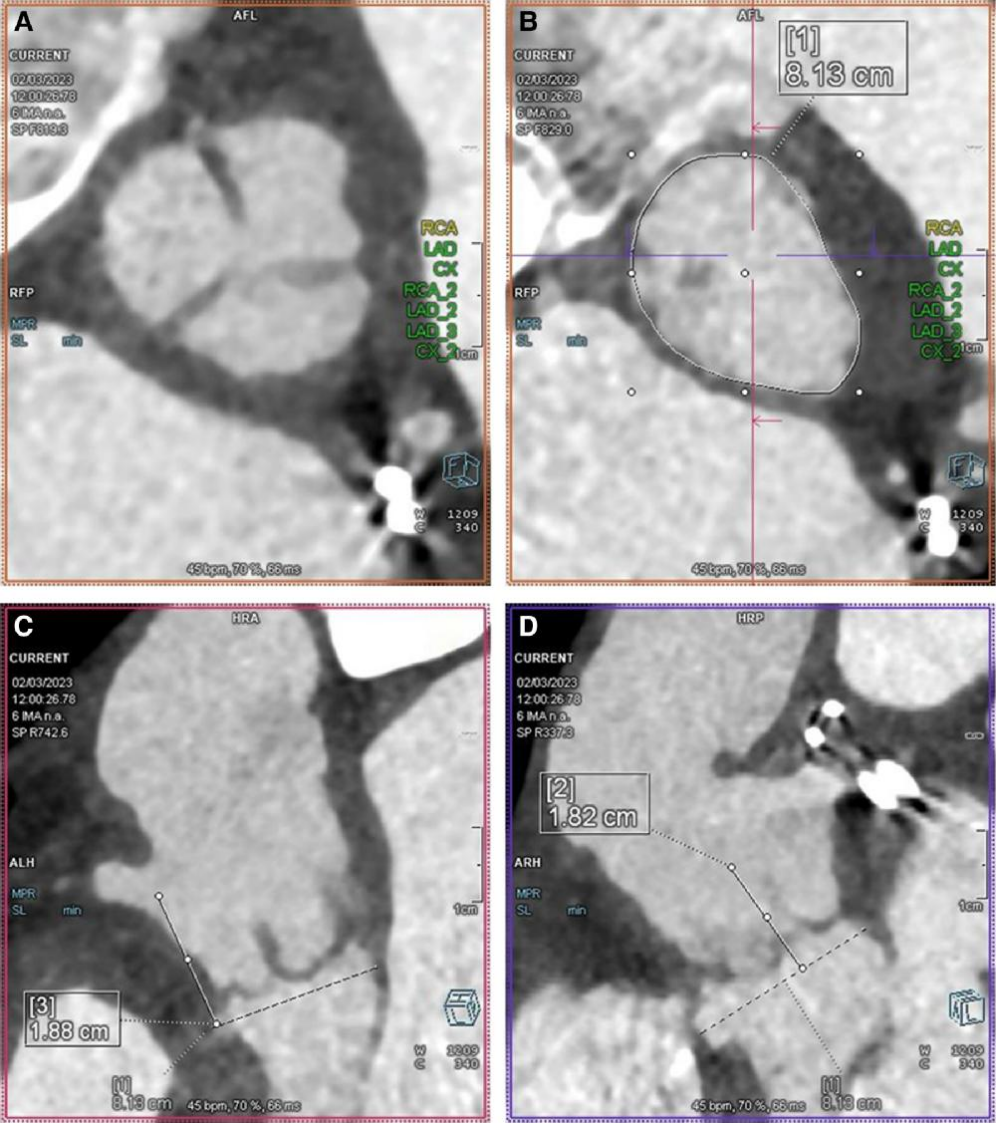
Computed tomography scan showing absence of leaflet calcification (*A*), a perimeter of 8.13 cm (*B*), right coronary artery height of 18.8 mm (*C*), and left main coronary height of 18.2 mm (*D*).
